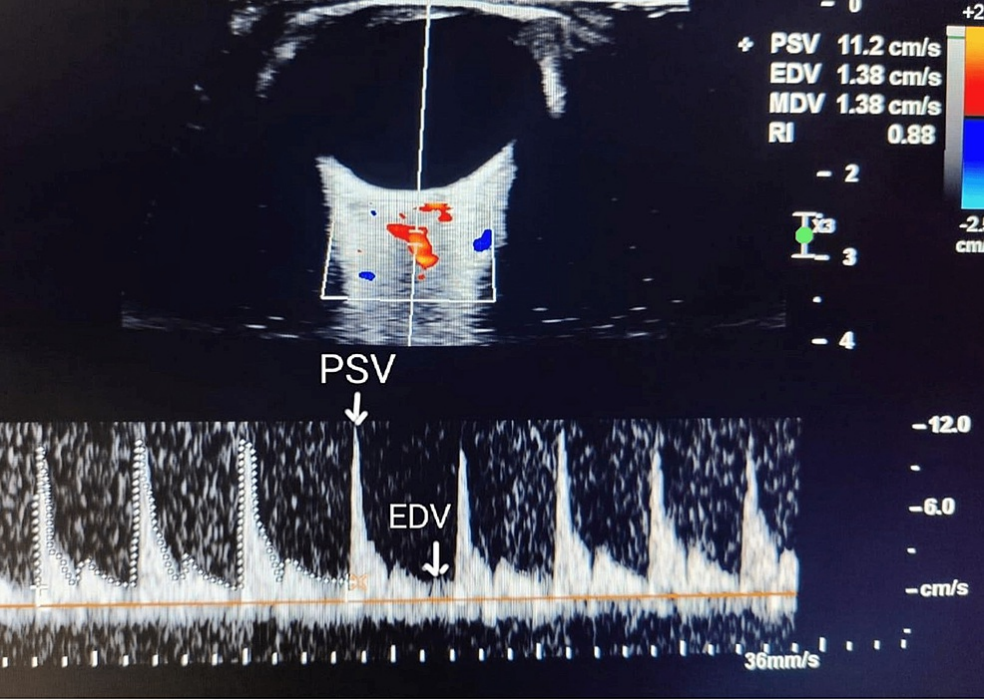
Ophthalmic artery Doppler waveform. PSV: peak systolic velocity, EDV: end diastolic velocity, MDV: mean diastolic volume, RI: resistive index
